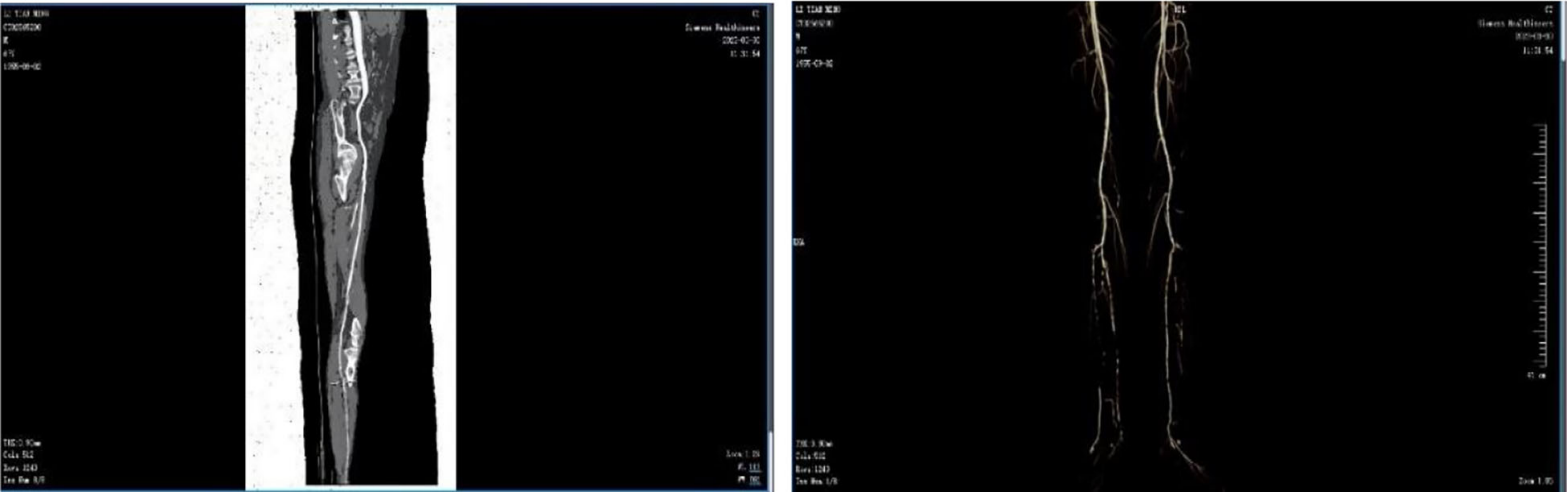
CTA showing irregular arterial walls with multiple patchy opacities and an irregular lumen. There was moderate to severe segmental stenosis in both the femoral and popliteal arteries, with severe stenosis in the proximal left profunda femoris. The anterior tibial, posterior tibial, and peroneal arteries were bilaterally occluded, with low-density opacities occurring in the posterior tibial arteries.
